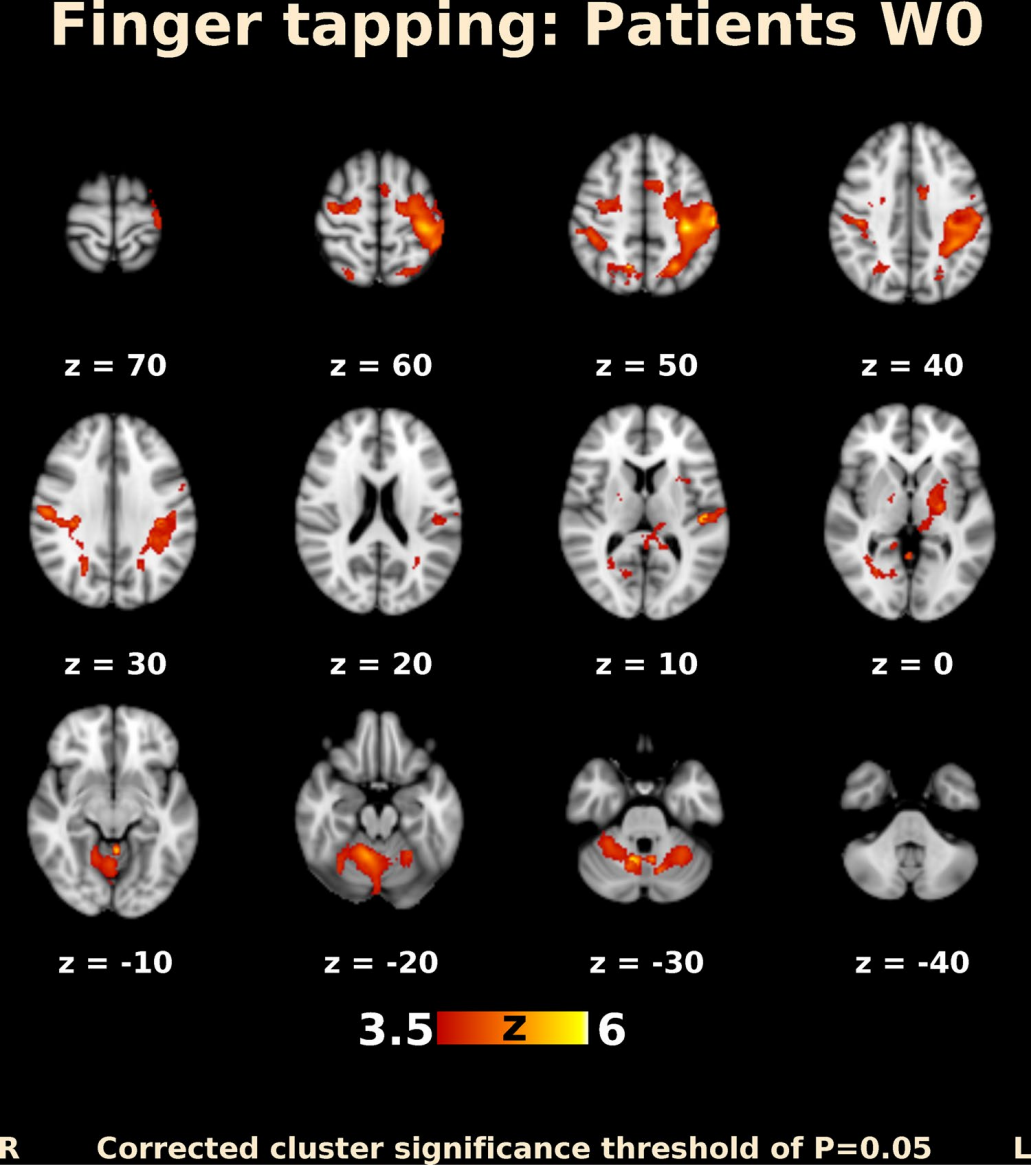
Functional MRI activation map in patients with CD before BoNT-A injection. Slices are labeled with *Z*/*Y* coordinate in standard MNI152 space
